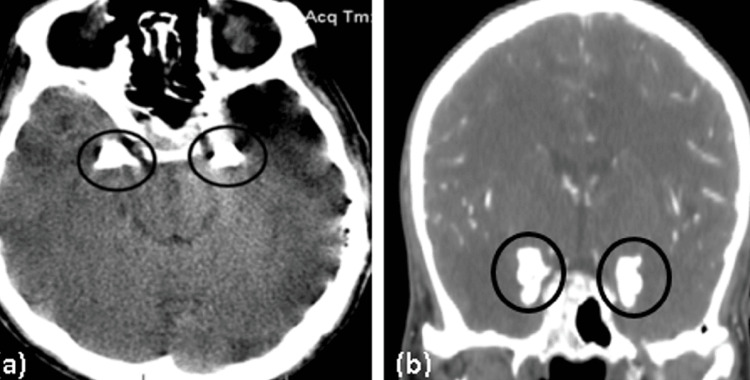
Axial non-enhanced (a) and coronal enhanced CT scan of the brain (b) demonstrating bilateral calcifications at the mesial temporal lobes (black circles) CT: computed tomography
